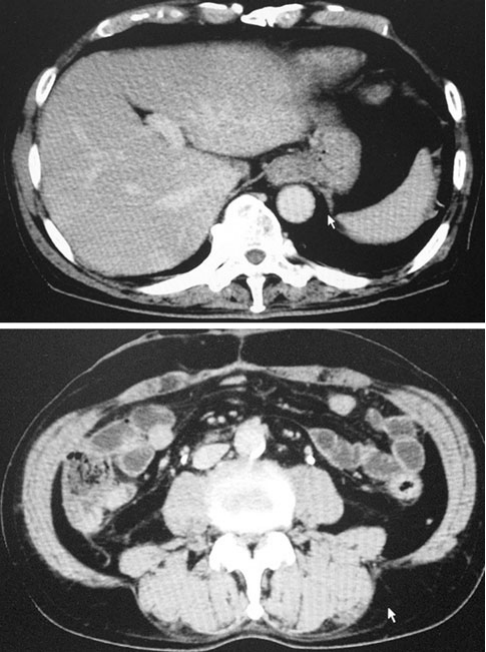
Abdominal computed tomography performed 7 weeks after the surgery. No ascitic fluid was seen in the abdomen
